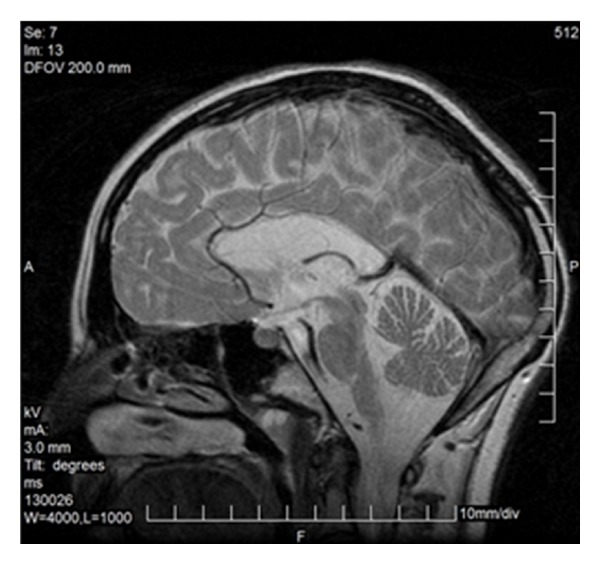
FSE, T2WI, sagittal plane. Leukoencephalopathy with vanishing white matter—corpus callosum is present but demyelinated to such an extent that practically indistinguishable from the cerebrospinal fluid on T2-weighted images.
